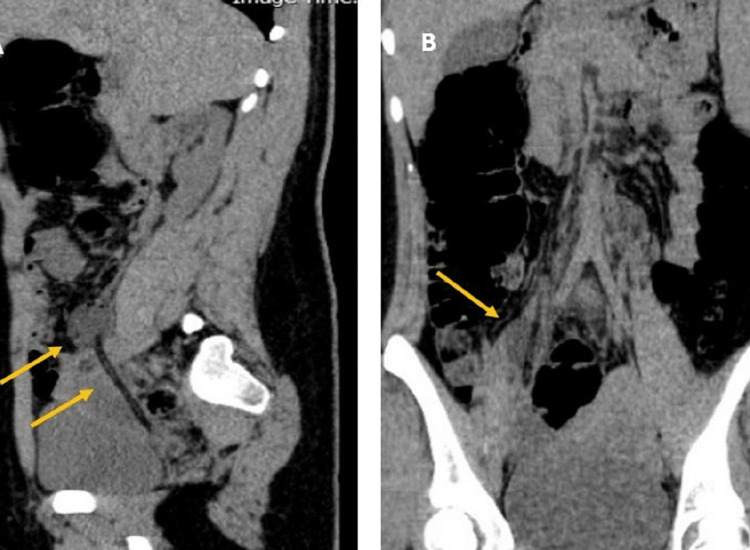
CT scan without contrast findings Tip of urinary catheter in the middle part of the ectopic ureter on the right side in sagittal (A) and coronal (B) planes CT: Computed Tomography
